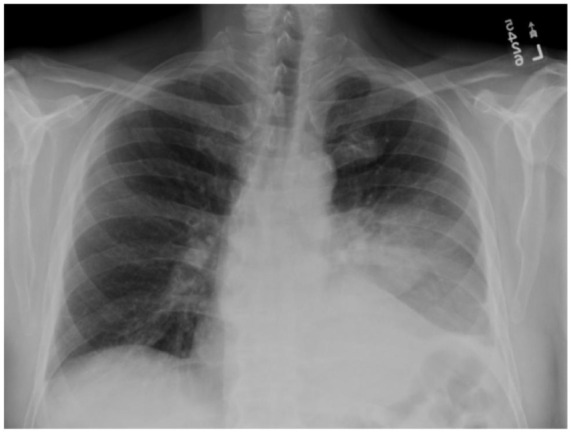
Initial CXR in the hospital showing left lung airspace opacities suspicious for CAP. CXR: Chest radiography; CAP: Community-acquired pneumonia.
